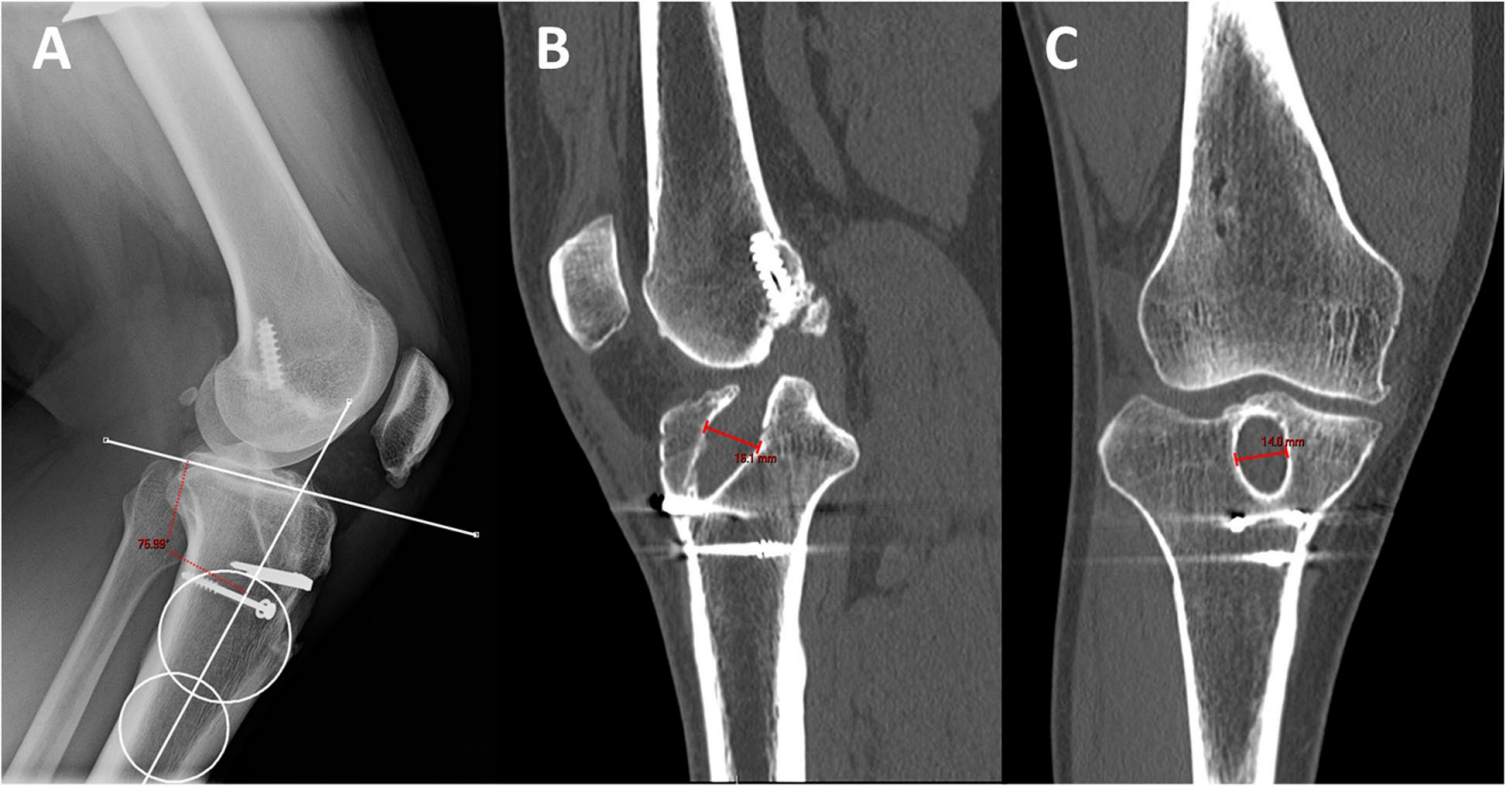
Preoperative radiograph and CT scan slices showing posterior tibial slope of 14 degrees (A) with tunnel widening of 16 mm on the sagittal (B) and 14 mm on the coronal (C)
